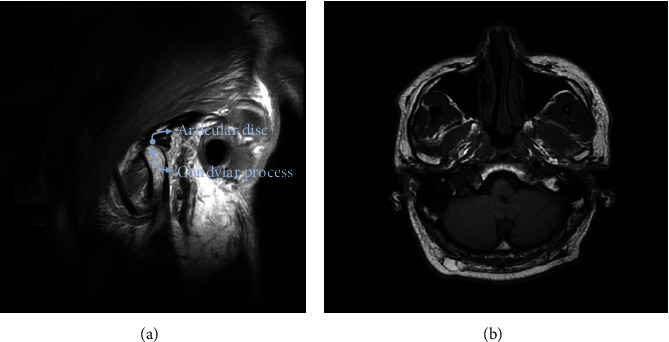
Magnetic resonance imaging (MRI) of the temporomandibular joints (TMJs). (a) T2-weighted sagittal MRI indicates that the anatomical structure of the TMJ region is normal. (b) T2-weighted axial MRI shows no pathologic changes in the TMJ region.
